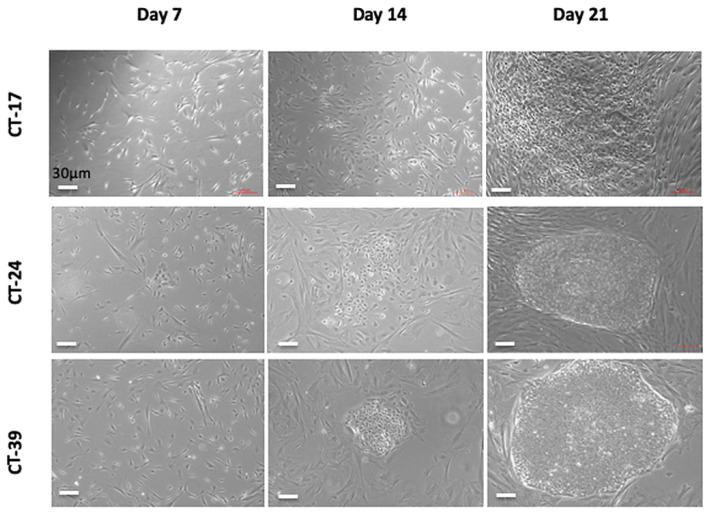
Observed changes to the CT-MSC during reprogramming. The CT-MSCs’ transition throughout the 21 days of reprogramming. Colonies present with a human embryonic stem cell-like morphology with defined boarders and a high ratio of nucleus to cytoplasm. The colonies were then picked and expanded for further characterization. Scale bar = 30 µM for all panels.
